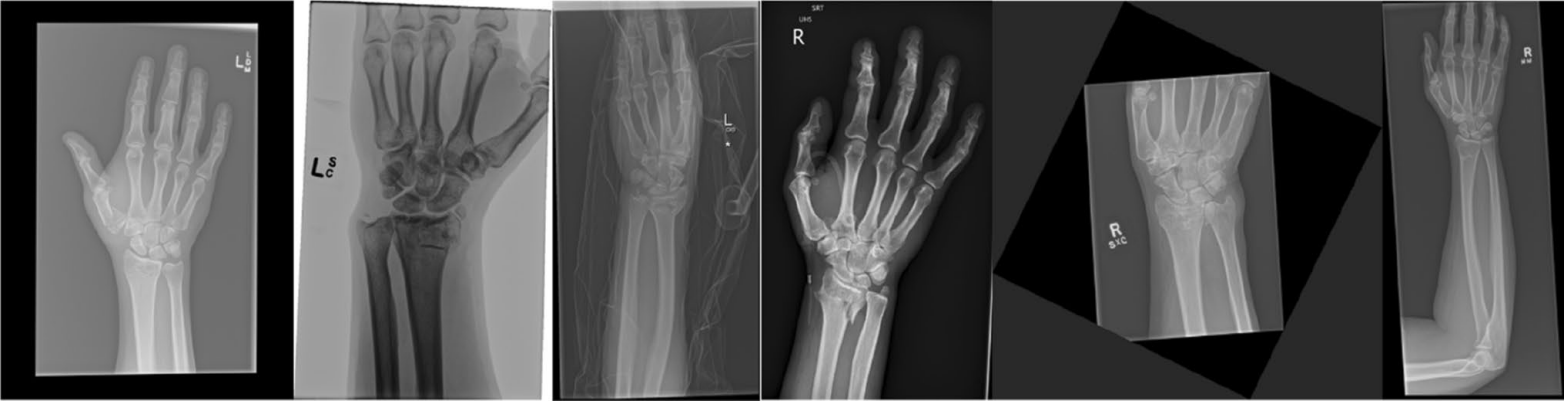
X-ray image examples from the dataset used in this study
